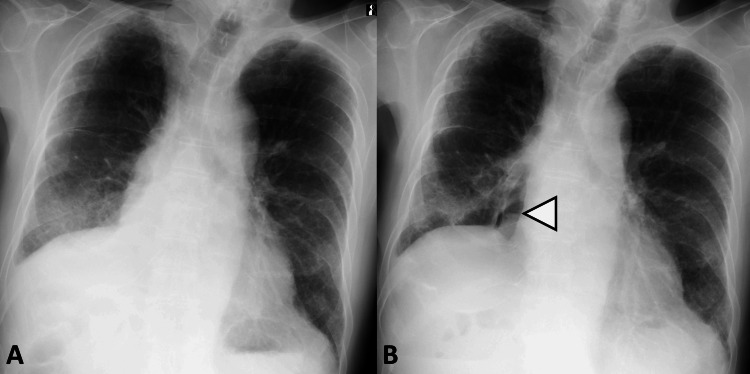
Chest radiographs after right lower lobectomy (A) Chest radiograph obtained at the second outpatient visit one month after discharge showing excellent lung expansion. (B) Chest radiograph one week later showing the air-fluid level and hyperlucency in the right lower lung field (arrowhead).
